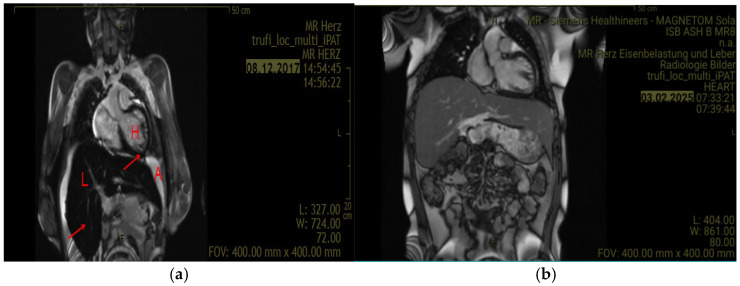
Coronal reconstructed T2*-weighted MRI images demonstrating myocardial and hepatic iron overload before and after iron chelation therapy. (a) The left panel shows diffuse hypointense signal (dark areas) in the liver and myocardium, consistent with iron deposition. (b) The right panel shows a follow-up MRI performed 7 years later, demonstrating improvement in iron burden (less hypodense signal) following iron chelation therapy. Abbreviations; H = heart, L = liver, Arrow = iron deposits (hypointense (dark) signals), A = ascites.
